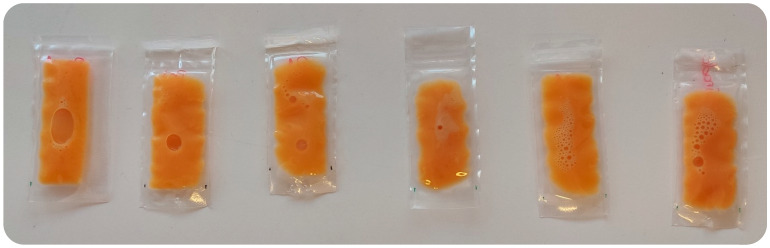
Images of whole egg samples packaged after each treatment. From left to right: raw whole egg, whole egg treated by commercial thermal pasteurization (60 °C/3.5 min), shorter thermal pasteurization (60 °C/1.75 min), moderate pressure (160 MPa/5 min), ultrasound (50% amplitude/1 min), and combined treatment (160 MPa/5 min–50% amplitude/1 min–60 °C/1.75 min).
